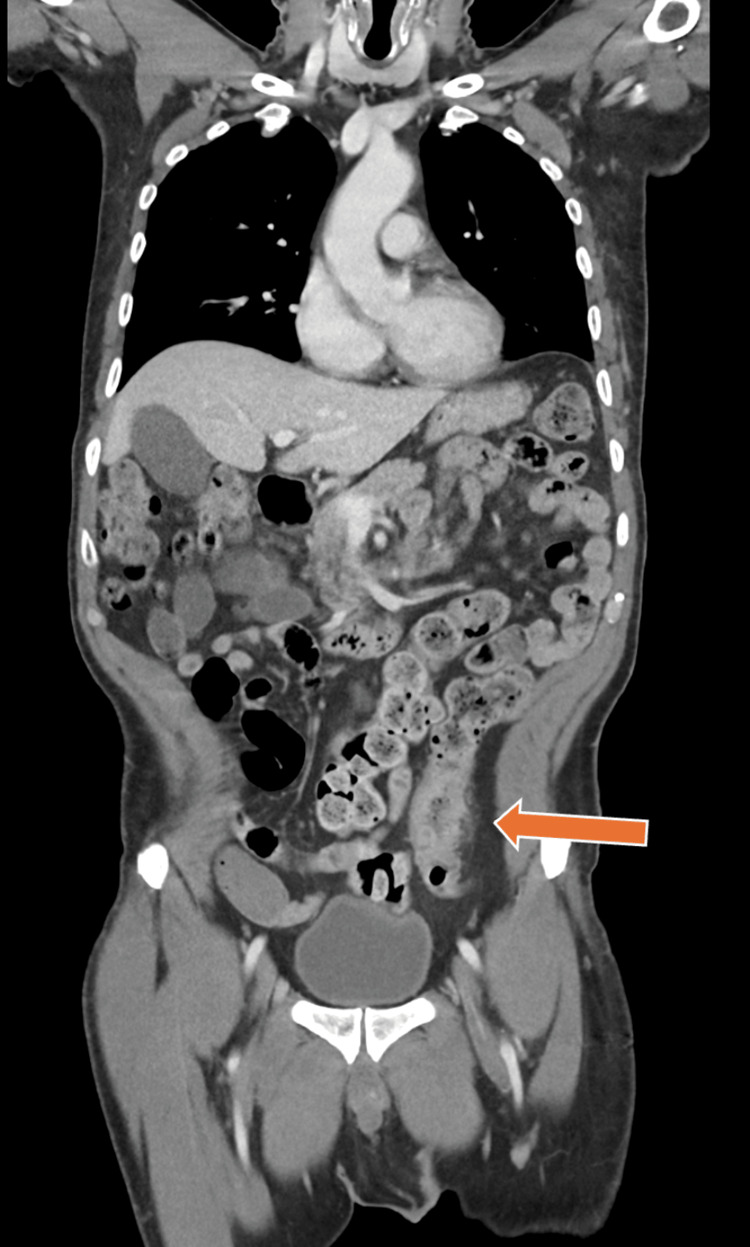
Contrast-enhanced CT of the abdomen and pelvis demonstrating segmental wall thickening and mucosal hyperenhancement of the distal descending and sigmoid colon (arrow), with surrounding inflammatory changes, consistent with active colitis, without evidence of free intraperitoneal air or perforation.
